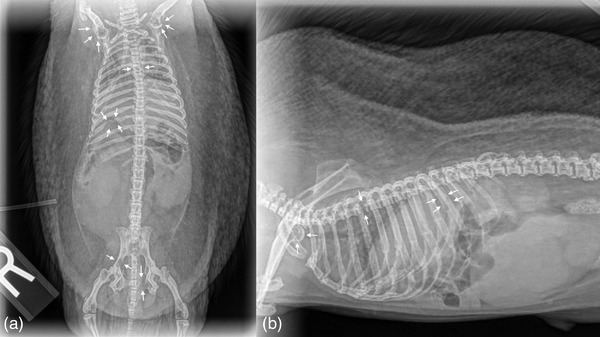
Follow‐up ventrodorsal (a) and right lateral (b) full body radiographs of the hedgehog. There are variably sized lytic lesions within the proximal humeral metaphyses and humeral heads, the body of T5, right ileum, left pubis and ribs (most prominent at the right 11th rib)
